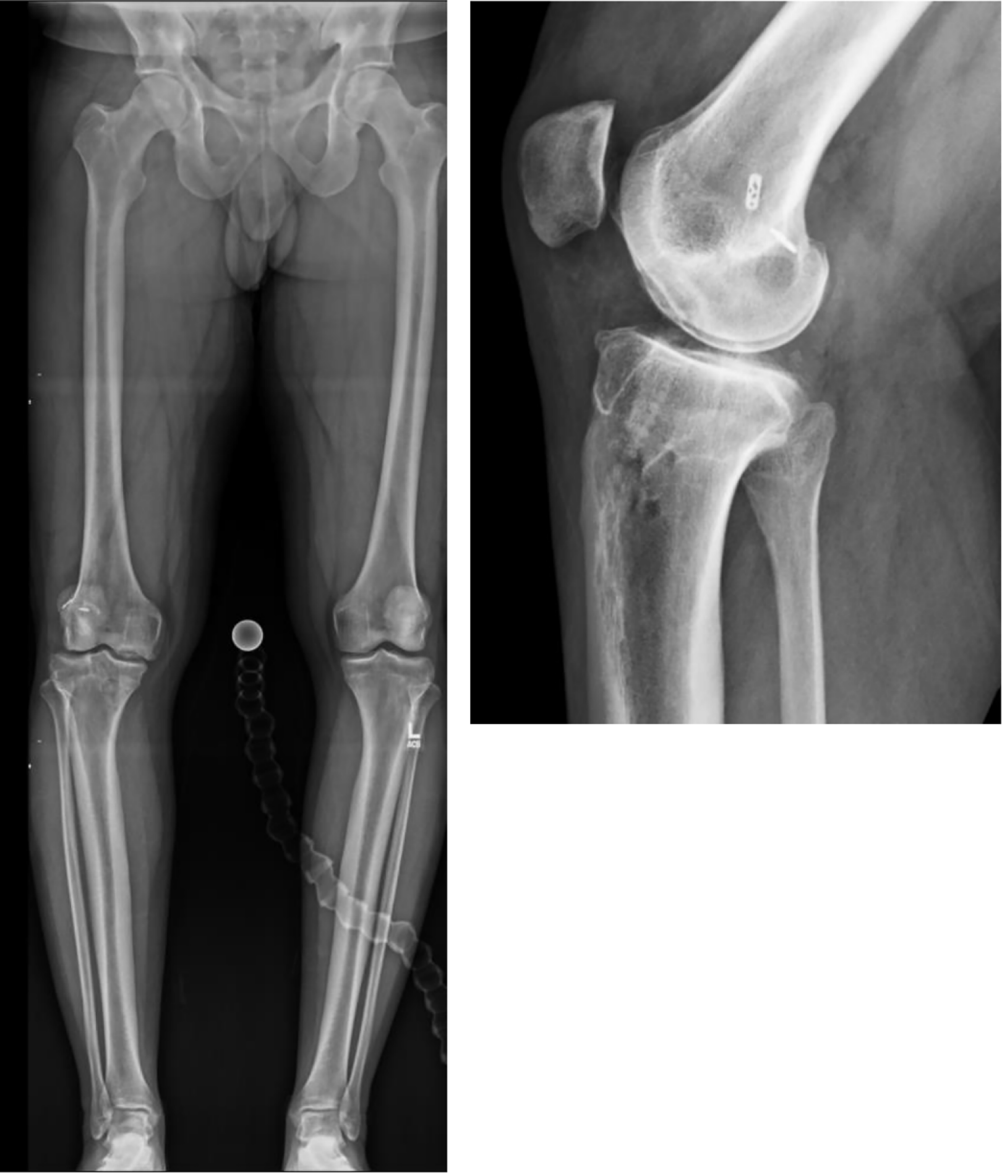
Full-length anteroposterior mechanical axis view and a knee lateral radiograph of a 25-year-old patient with right knee in varus alignment with excessive tibial slope, with a history of ACL reconstruction with allograft and meniscal repair, complicated by graft rupture, ACL tunnel bone grafting and osteochondral allograft to his medial femoral condyle, revision ACL reconstruction with hamstring autograft, and a subsequent re-rupture
